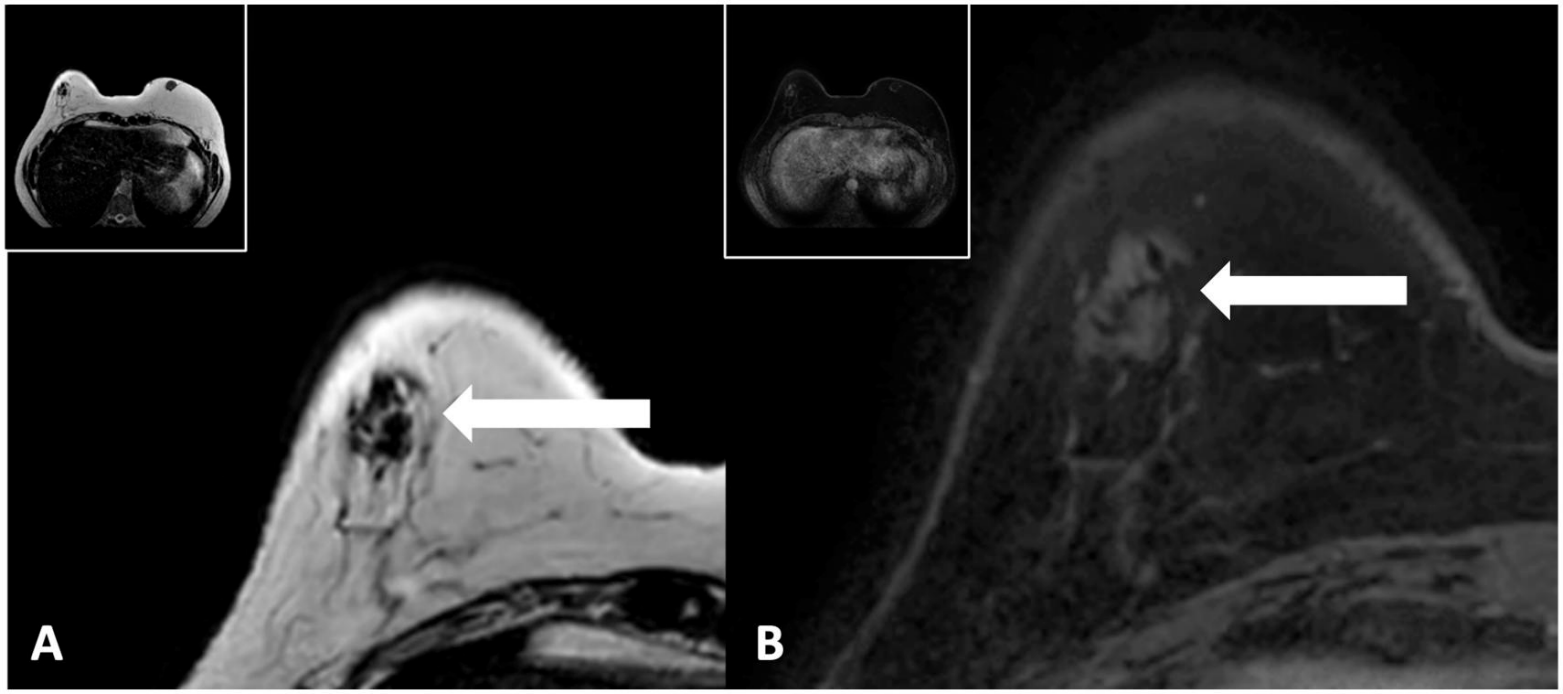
Typical aspects of fat necrosis on MRI. The images are from a 46-year-old female patient that underwent breast MRI 2 years and 10 months after her last AFT. Detailed images of the right breast demonstrate a T2 hypointense lesion (or mass) in the upper-outer quadrant of the right breast (A) without any enhancement on the T1W-sequence after contrast administration (B). Abbreviation: AFT = autologous fat transfer.
